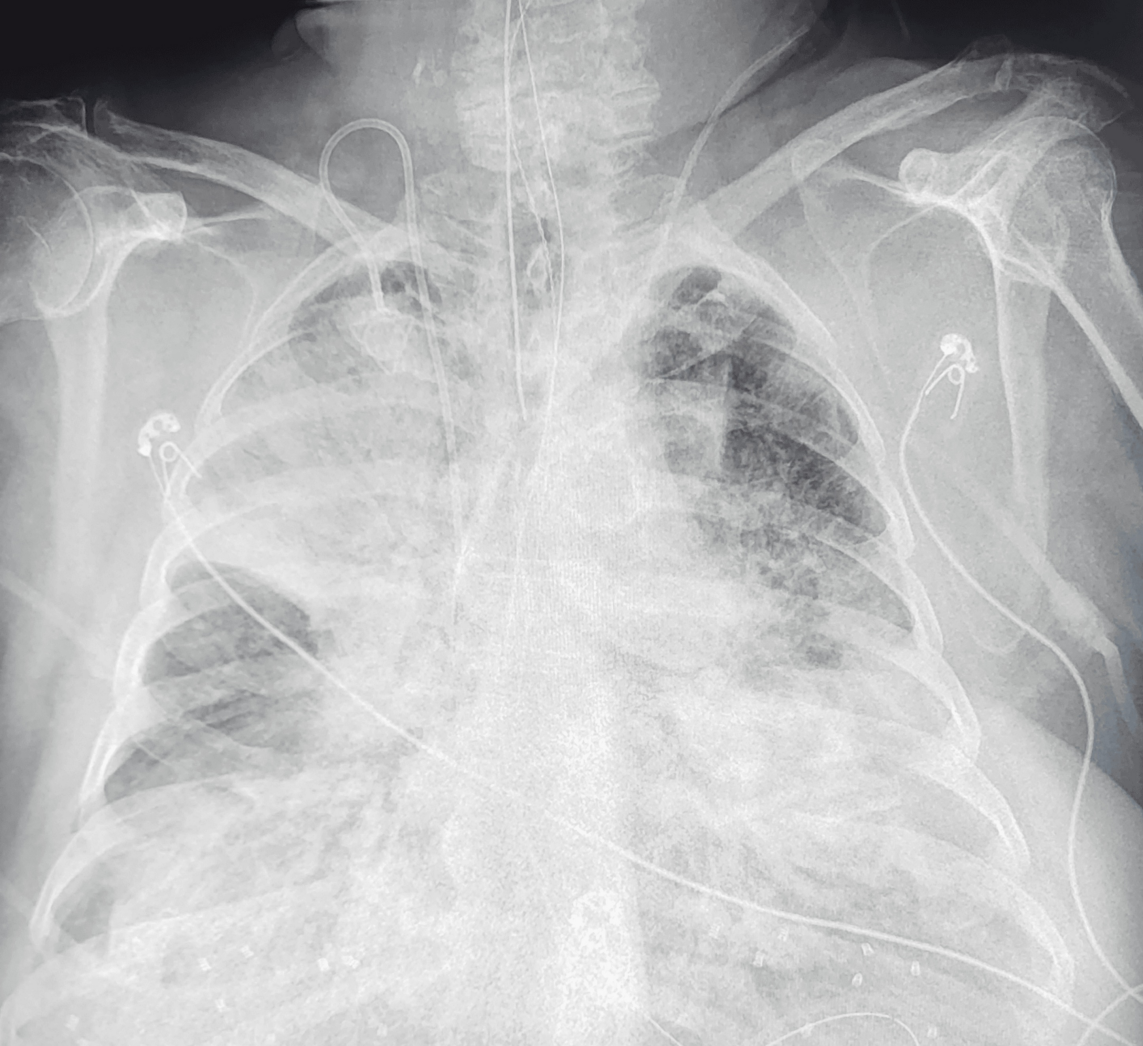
Chest X-ray demonstrating bilateral diffuse infectious pneumonia.
